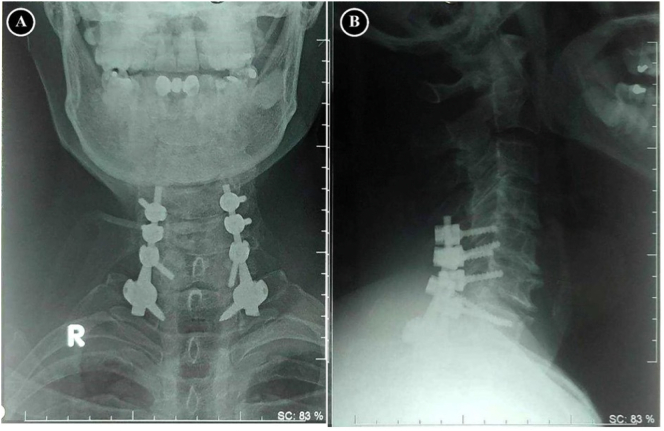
Postoperative radiography showed the realignment of the cervical spine in (A) Anterior View and (B) Lateral View, which stabilized using two lateral mass screws (C5-C6) and pedicle screws (C7-T1).
